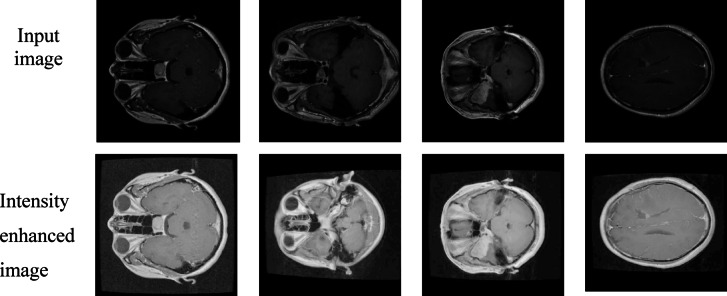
Brain MRI images after log transformation for intensity enhancement.
